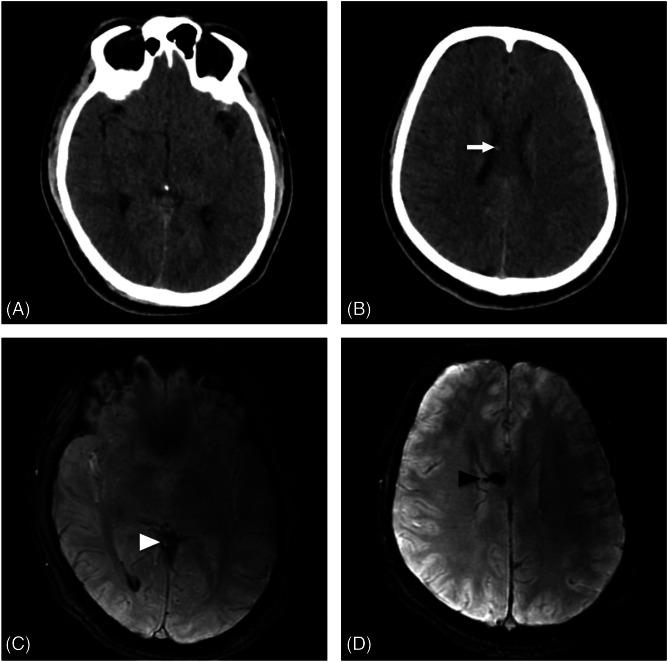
Non-contrast computed tomography brain of a 24-year-old male (axial view): a) without any evidence of Intraparenchymal injury; b) showing tiny hemorrhagic foci (white arrow). Magnetic resonance imaging of the brain (axial 3-D susceptibility-weighted angiography) at the corresponding section showed; c) blooming foci in the posterior horn of the right lateral ventricle (white arrowhead) suggestive of minimal intraventricular hemorrhage; d) blooming foci in the body of corpus callosum on the right side (black arrowhead), measuring 6.0 × 8.0 mm—likely minor hemorrhagic contusion.
